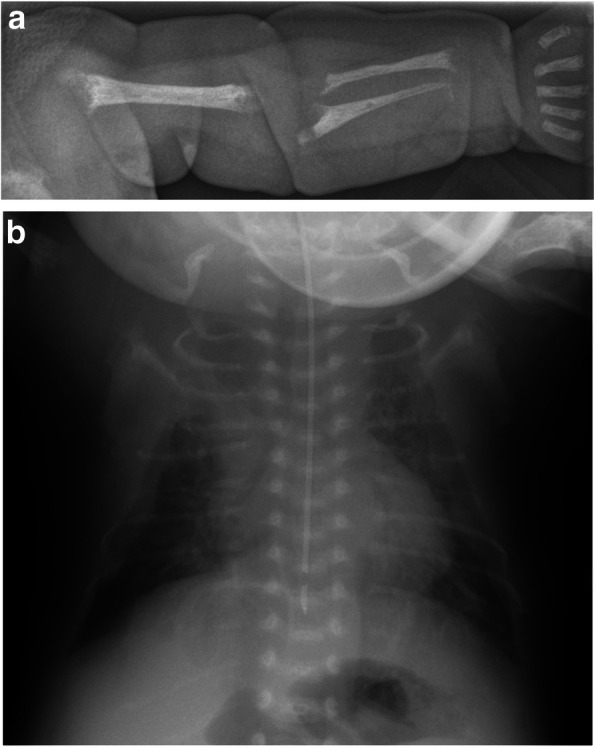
Representative radiographs (Patient 1 at age 4 weeks). a Radiograph of the left upper limb showing severe rickets (bold arrows) and tongue-like metaphyseal lucencies (dotted arrows). b Radiograph of the chest showing gracile undermineralized ribs, a narrow thoracic cavity and small lung volumes
